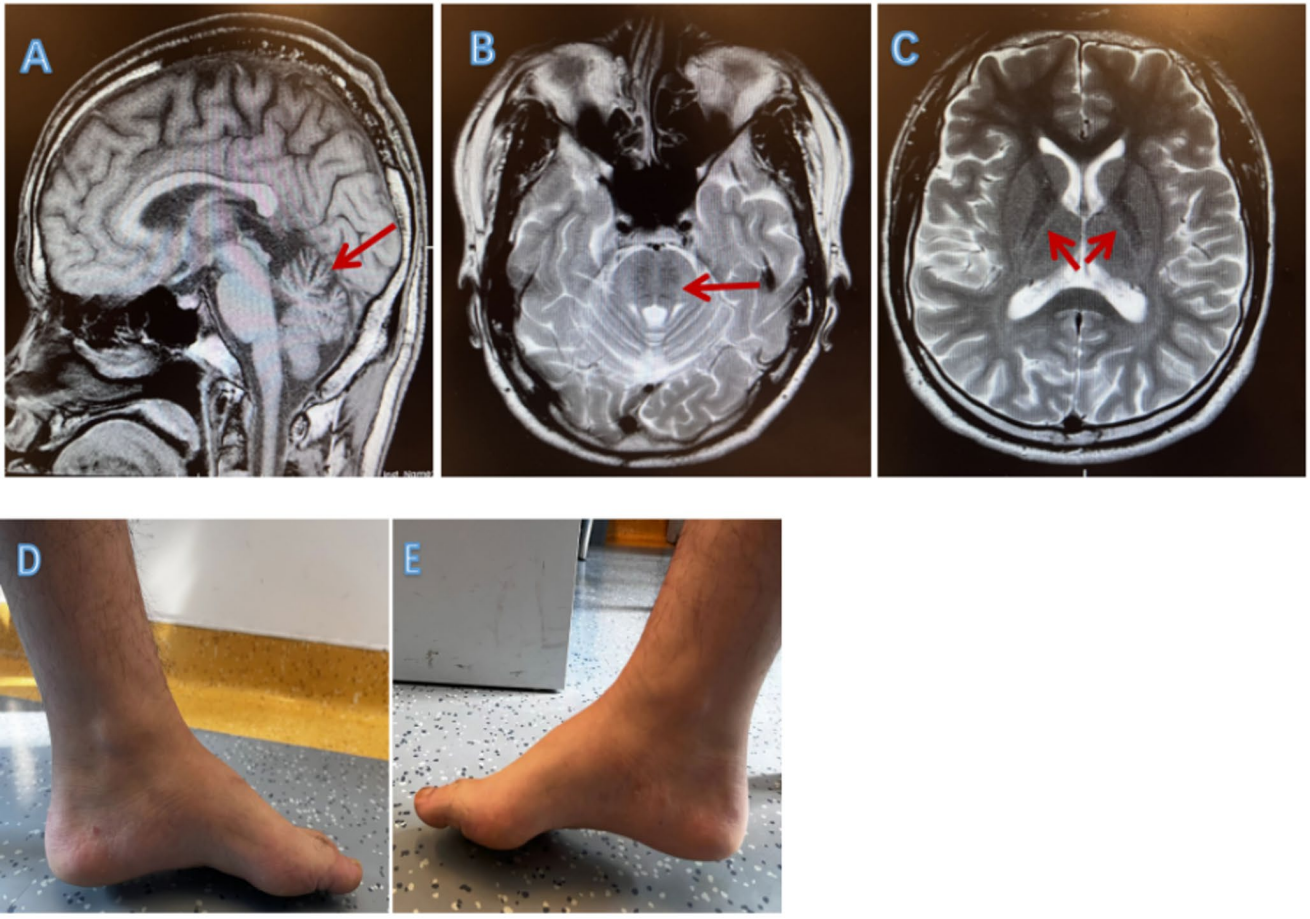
Clinical and neuroimaging characteristics of the patient. (A) Atrophy of the cerebellar vermis on the sagittal T2-weighted image (red arrows); (B) Cross-sectional T2-weighted image showing pontine hypointensity (red arrows); (C) T2 hyperintense margins around the thalamus on either side of the cross-section (red arrows); (D–E) pes cavus and hammertoes in patient
